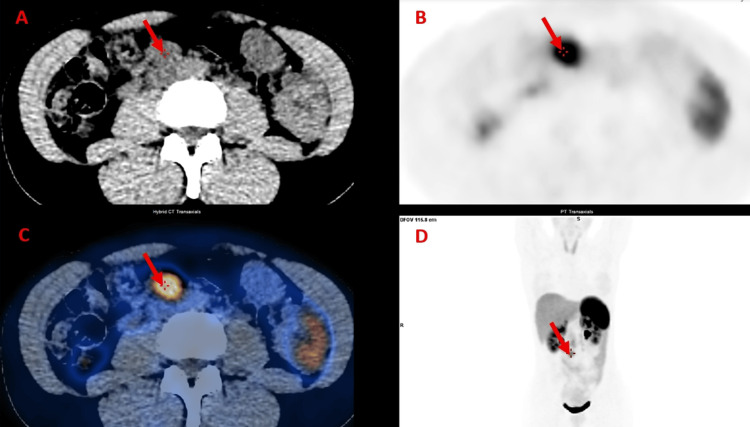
DOTATOC PET/CT demonstrating a hypermetabolic retroperitoneal nodule consistent with paraganglioma Axial CT (A), axial ^68^Ga-DOTATOC PET (B), fused ^68^Ga-DOTATOC PET/CT (C), and coronal ^68^Ga-DOTATOC PET (D) images show a solitary 25mm hypermetabolic retroperitoneal nodule (red arrow), located in the right para-aortic region. The lesion demonstrates intense radiotracer uptake consistent with somatostatin receptor overexpression, supporting the diagnosis of paraganglioma. No additional sites of abnormal uptake are identified.
